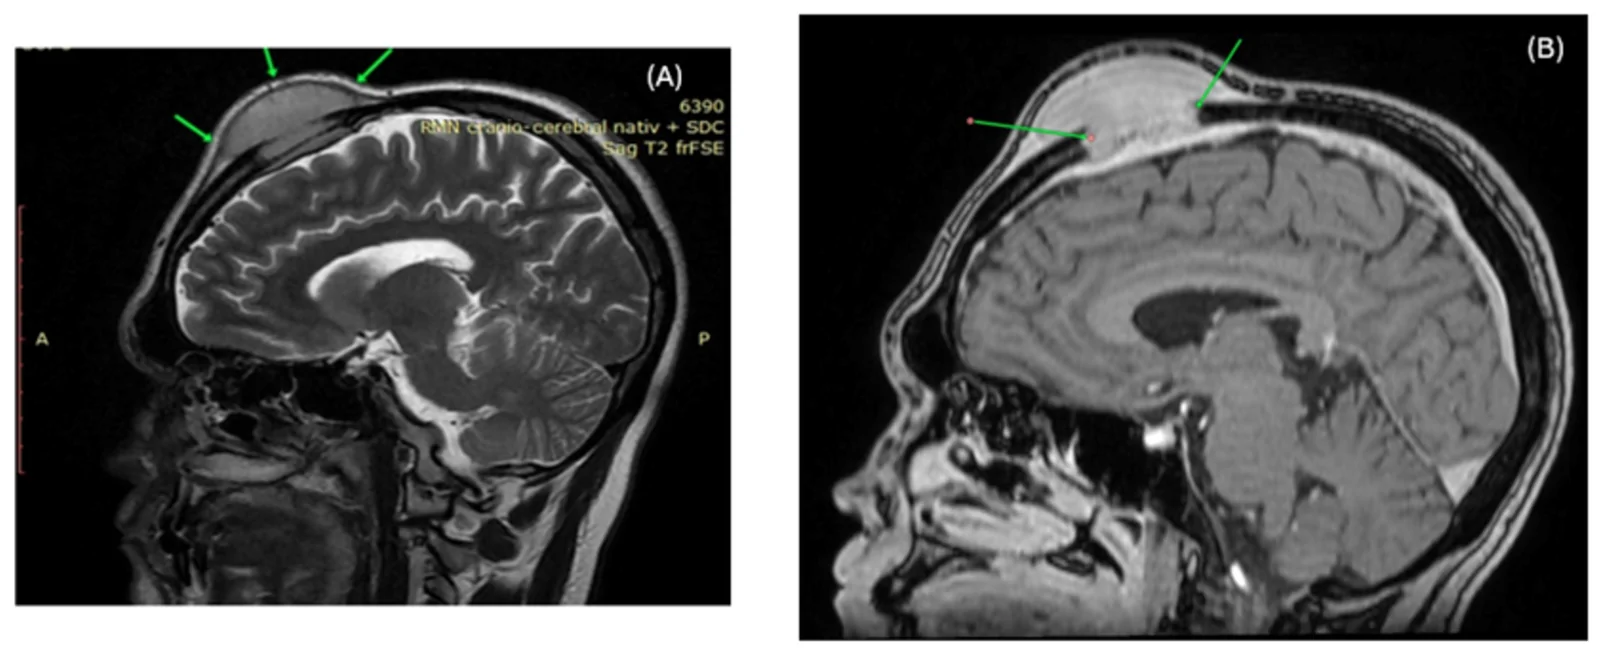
Brain MRI showed a frontal osteolytic bone tumor extended epicranially and subdurally (A) with significant contrast uptake, infiltrating the superior sagittal sinus (B) but without brain parenchymal involvement. The letters A and P indicate anterior and posterior orientation.
